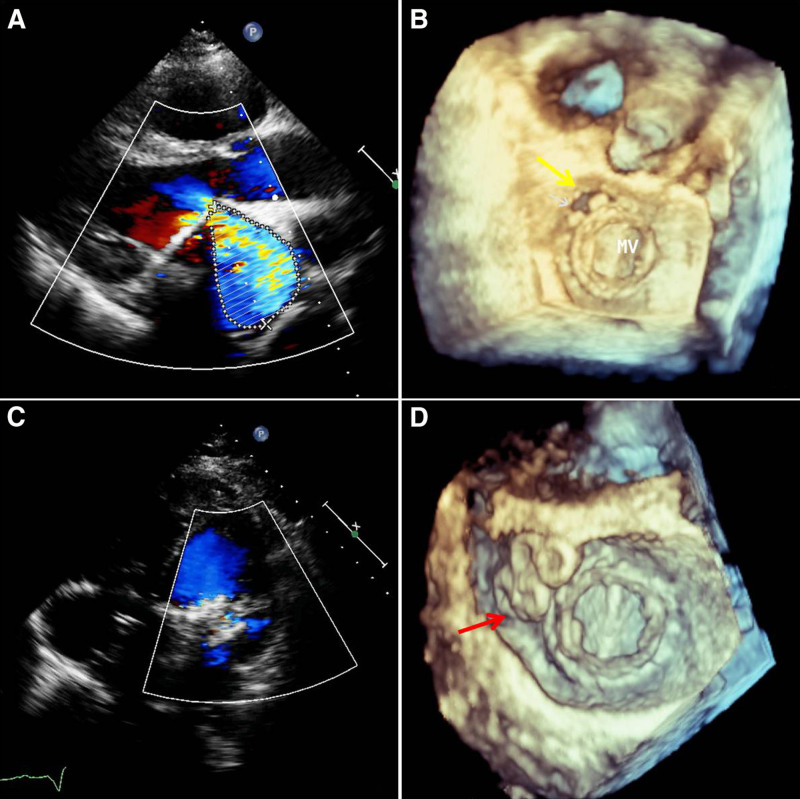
Echocardiography before the procedure and during the follow-up period. (A) 2-dimensional transesophageal echocardiography shows the mitral PVL before the procedure; the color jet shows the PVL regurgitation. (B) 3-dimensional transesophageal echocardiography shows the mitral PVL before the procedure. (C) 2-dimensional transesophageal echocardiography shows the occluder and no residual regurgitation during the follow-up period. (D) 3-dimensional transesophageal echocardiography shows the mitral PVL closed with the occluder (the *yellow arrow* shows the PVL. The *red arrow* shows the occluder). PVL = percutaneous paravalvular leak.
